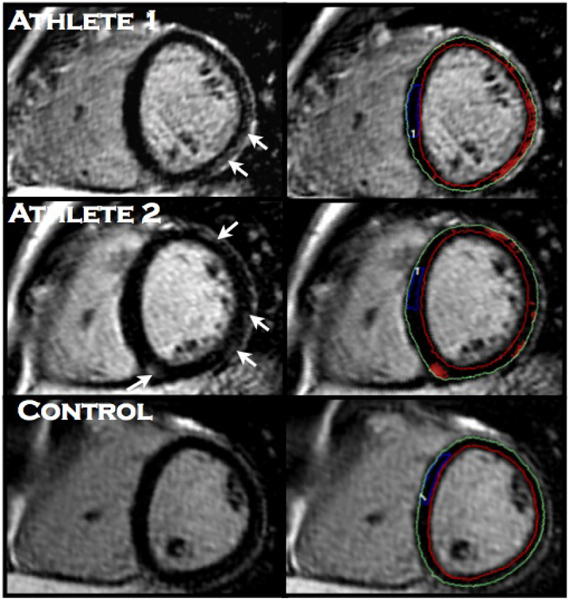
T1-weighted late gadolinium enhancement images. *Left panels*: Evidence of subepicardial non-ischemic myocardial fibrosis in athletes 1 and 2 (arrows), with none in healthy control. *Right panels*: Quantification of myocardial fibrosis by manually tracing a region of interest in health remote myocardium (blue outline). Automated computer thresholding set at 5 standard deviations above the mean signal intensity of remote myocardium detects fibrosis (red overlay). The detected extent of fibrosis for athlete 1 is 14.12%, 11.30% for athlete 2 and 0.20% for control.
